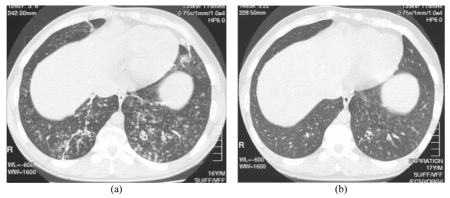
HRCT images of the lower chest in a 16 year-old boy (Case 2) at presentation (A), and 8 weeks later after a 6-week course of erythromycin 250mg twice daily. The bilateral bronchiectasis and prominent centri-lobular nodules with a tree-in-bud pattern shows marked improvement on the follow-up scan
